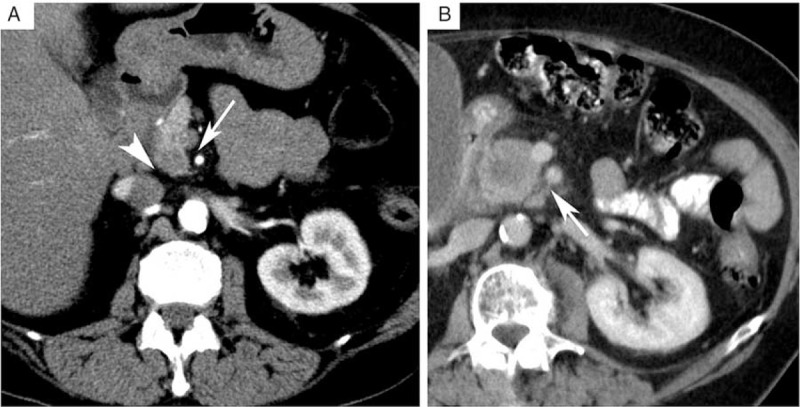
Assessment of radiologic parameters. A, Preoperative contrast enhanced multiphasic multidetector computed tomography (MDCT) demonstrating a normal *fatty tissue sheath* separating the superior mesenteric artery (SMA, arrow) and the inferior caval vein (ICV, arrowhead) from the pancreas. A small hypovascular/hypodense tumor in the pancreatic head can be seen. B, Preoperative contrast enhanced MDCT demonstrating the presence of stranding, that is increased attenuation, in the SMA fatty tissue sheath (arrow).
